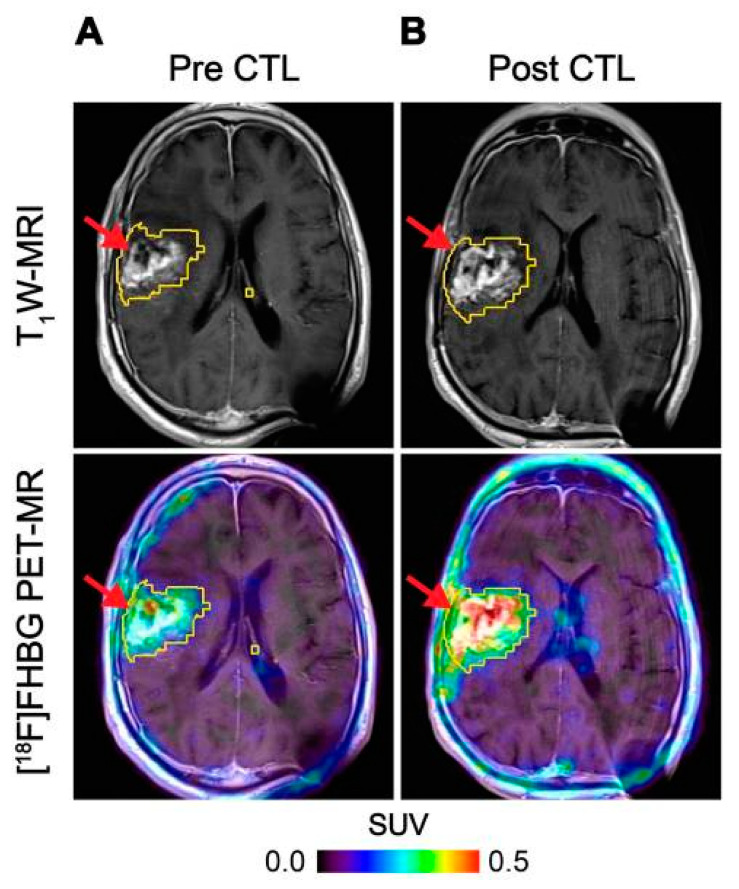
Imaging of HSV1-tk gene reporter expression in genetically modified CTLs with [^18^F]FHBG. [^18^F]FHBG-PET imaging was performed in a patient with recurrent glioblastoma multiforme tumour in the right frontoparietal lobe before (A) and 1 week after (B) CTL infusions. Allogeneic CTLs and IL-2 were injected intratumorally (red arrows). Tumour recurrence was monitored by T_1_-weighted (T_1_W) MRI (top panels) and [^18^F]FHBG-PET images were fused with the MR images (bottom panels). A significant increase in [^18^F]FHBG uptake and PET signal was detected following CTL infusion, which was likely to be due to CTL cell trafficking. Figure reproduced from [51].
